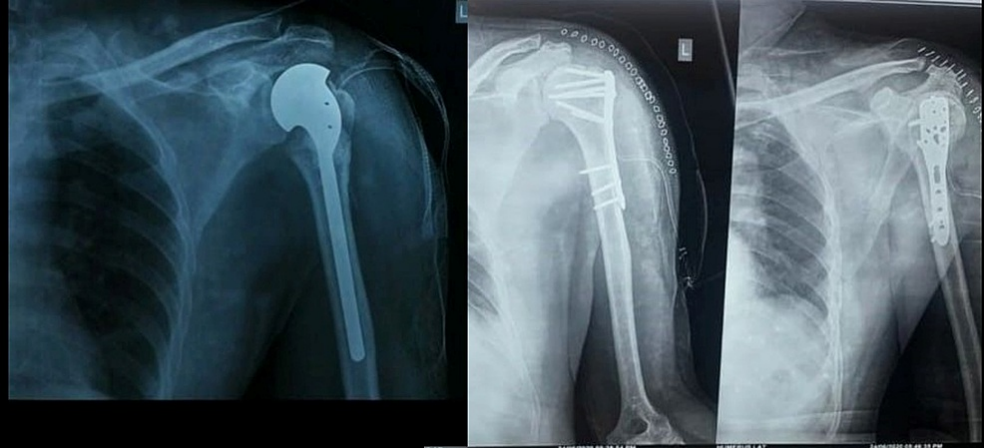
Immediate post-operative X-ray for Neer's hemiarthroplasty and proximal humerus internal locking system (PHILOS®) plating
